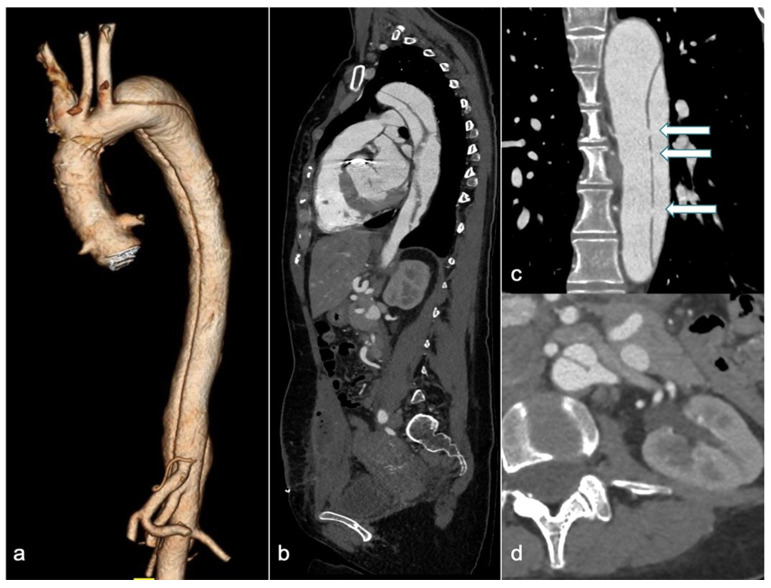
A 31-year-old woman with Marfan syndrome and a history of ascending aortic dissection, previously treated with a Bentall procedure, presented with chest pain a few days postpartum. CTA revealed an extension of the dissection into the descending thoracic and abdominal aorta (3D volume rendering in (a), and sagittal and coronal views in (b and c), respectively), with simultaneous enhancement of both the true and false lumens due to multiple intimal fenestrations (arrows in (c)). The sagittal view (b) shows a heterogeneous collection into the rectus abdominis muscles following a recent cesarean section. Axial image (d) reveals the left renal artery originating across both the true and false lumens. CTA: computed tomography angiography and 3D-VR: 3D volume rendering.
